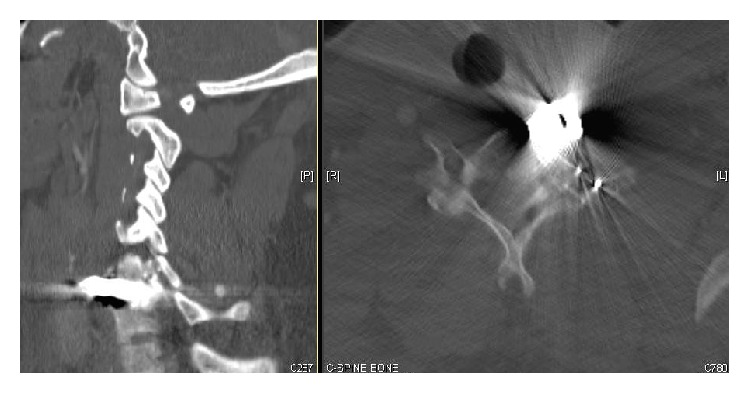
Cervical spine CT scan (sagittal and axial cuts) showing the bullet along with the C6 vertebral body and lateral mass fractures.
